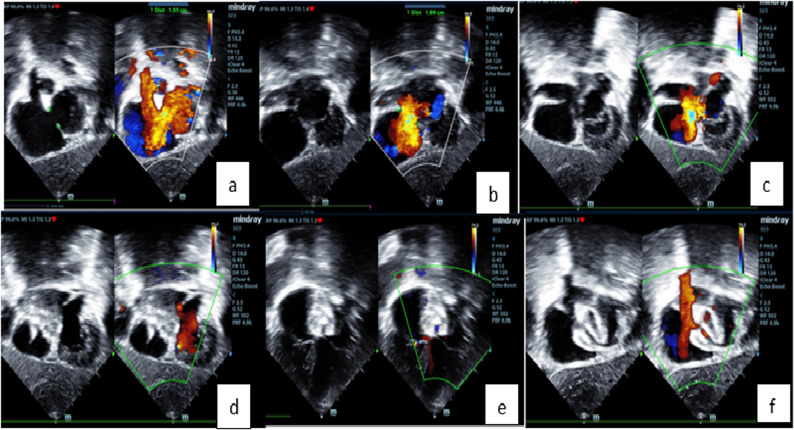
Transthoracic echocardiography–guided transcatheter ASD device closure using LADEDT. (a, b) shows ASD with left to right shunt in subcostal view using TTE with sheath inside LUPV. (c) shows introducing the device through the long sheath with left disc inside LUPV. (d) shows disengagement of the left disc with no flow detected by echo across ASD. (e, f) shows echo after device release in place with no residual flow
